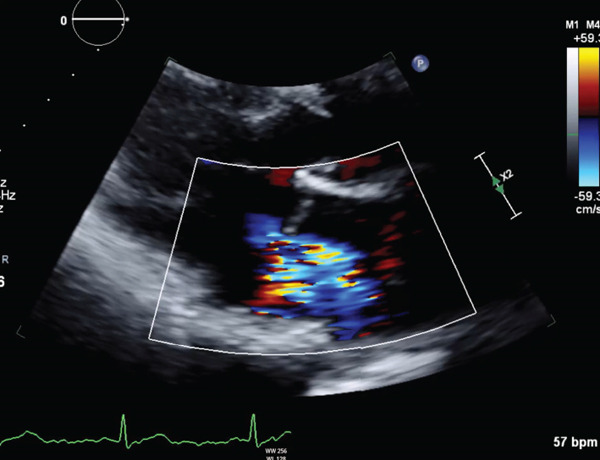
Color Doppler imaging demonstrates significant mitral regurgitation indicated by color flow.
